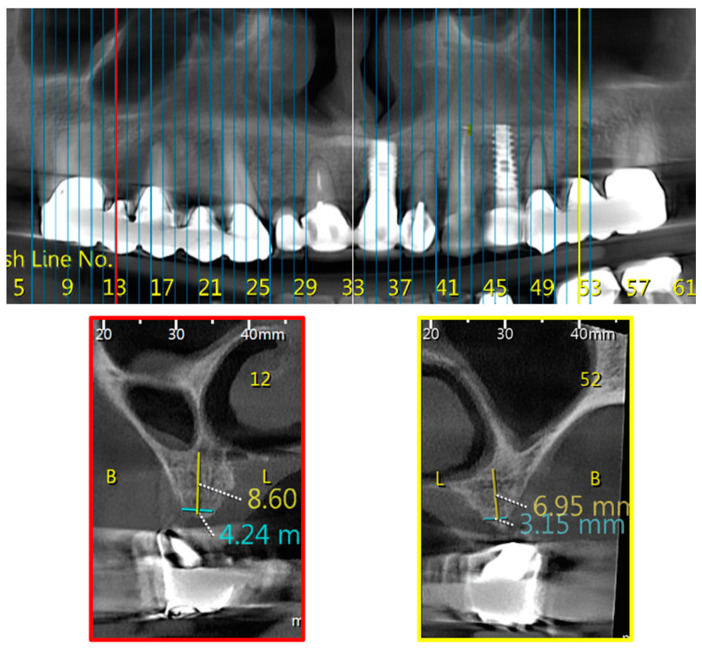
Bilateral Class 3; Upper part—CBCT panoramic reconstruction; Lower part—from right to left—CBCT cross sections: cross section number 52—demonstrate left nasal and maxillary sinus “border cross section”, which is located distal to the mesial edge of the 1st molar, defined as Class 3; cross section number 12—demonstrate right nasal and maxillary sinus “border cross section”, which is located distal to the mesial edge of the 1st molar, defined as Class 3. B, buccal; L, lingual.
